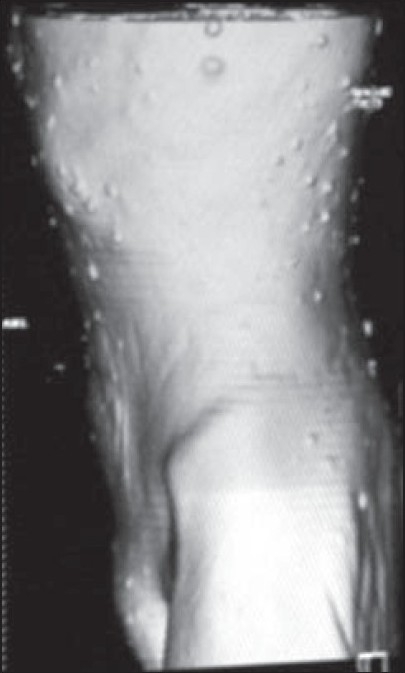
Surface-rendered CT image showing multiple cutaneous neurofibromas
